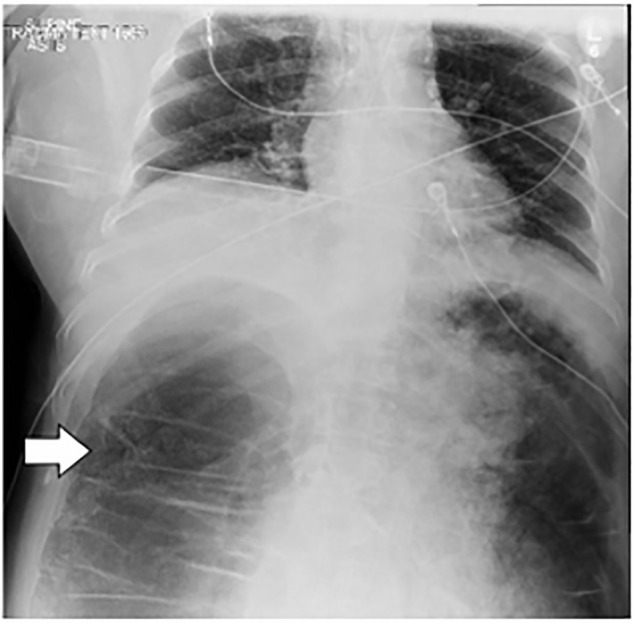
Supine chest radiograph after intubation demonstrating extremely dilated
